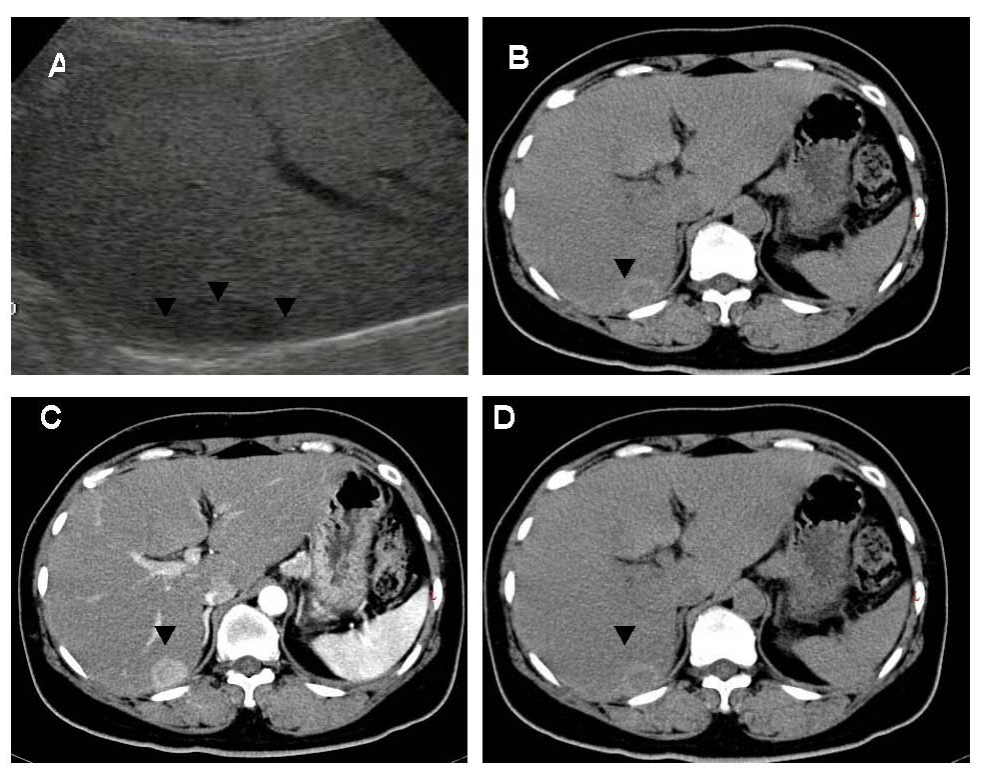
(A) Abdominal ultrasound shows a low echoic nodule in the sixth segment of the liver (arrowheads). (B) Noncontrast CT scan shows a well-circumscribed, low density nodule (arrowheads). (C) Dynamic CT scan shows enhancement of the nodule in the arterial phase (arrowheads). (D) Dynamic CT scan shows enhancement in the portal phase (arrowheads).
